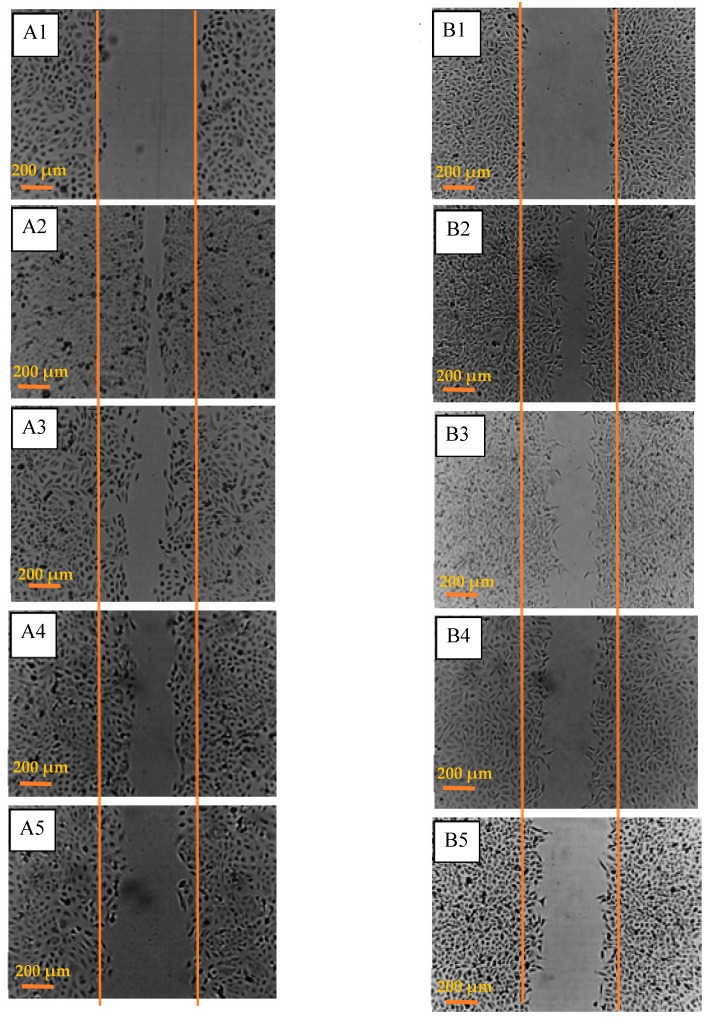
Effect of IR and/or AuNPs on cell motility in vitro. (A) DU145 (prostate) cancer cells; (A1) untreated controls at 0 h, (A2) untreated control at 24 h, (A3) cells exposed to 5 Gy X-rays after 24 h, (A4) cells treated with 1 mM AuNPs at 24 h and, (A5) cells treated with IR and AuNPs at 24 h and, (B) lung (A549) cancer cells; (B1) untreated controls at 0 h, (B2) untreated control at 24 h, (B3) cells exposed to 5 Gy X-rays after 24 h, (B4) cells treated with 1 mM AuNPs at 24 h and, (B5) cells treated with IR and AuNPs at 24 h.
